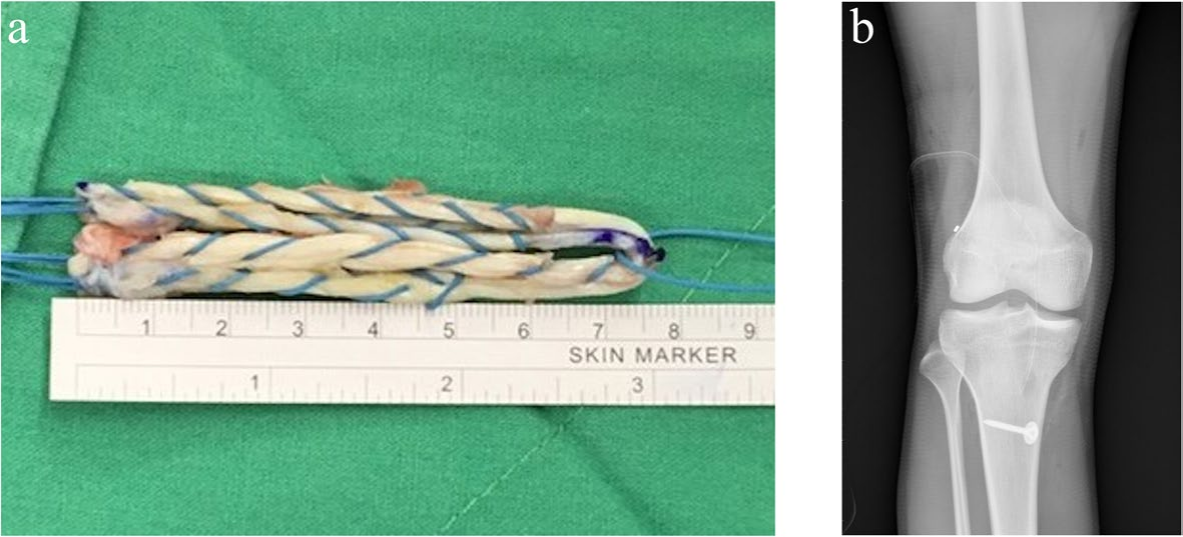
a Whipstitches at tibial ends of the graft with nonabsorbable sutures (No. 5 Ethibond) for later fixation with a cortical screw post. b Postoperative radiograph showing traditional hybrid tibial fixation using a bioabsorbable interference screw and cortical screw post
